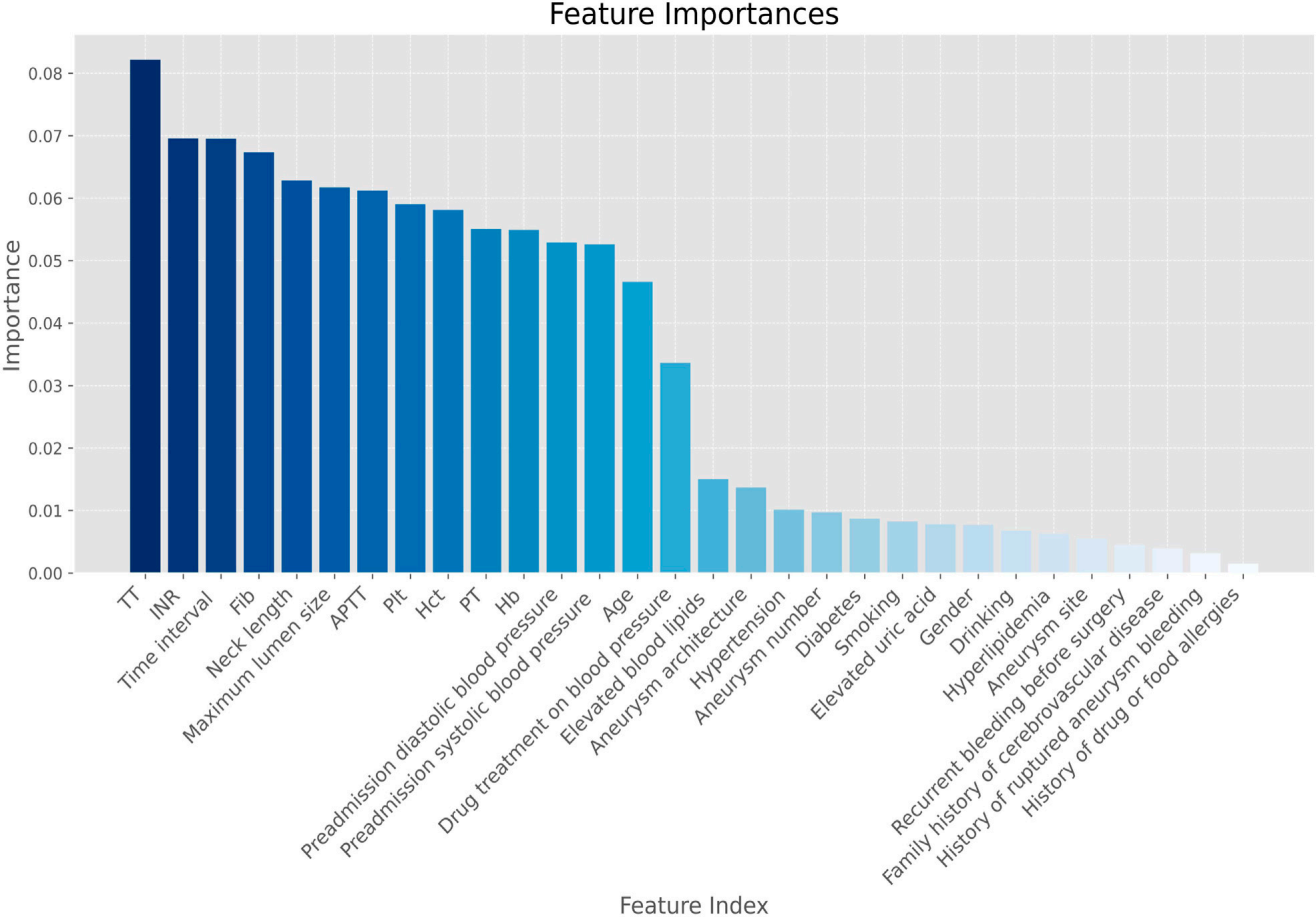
Feature screening importance plot. Note. Hb, hemoglobin; Hct, red blood cell specific volume; Plt, blood platelet; PT, prothrombin time; INR, international normalized ratio; APTT, activated partial thromboplastin time; Time interval, time interval between the onset of aSAH and the first-time CT examination; TT, thrombin time; Fib, Fibrinogen.
